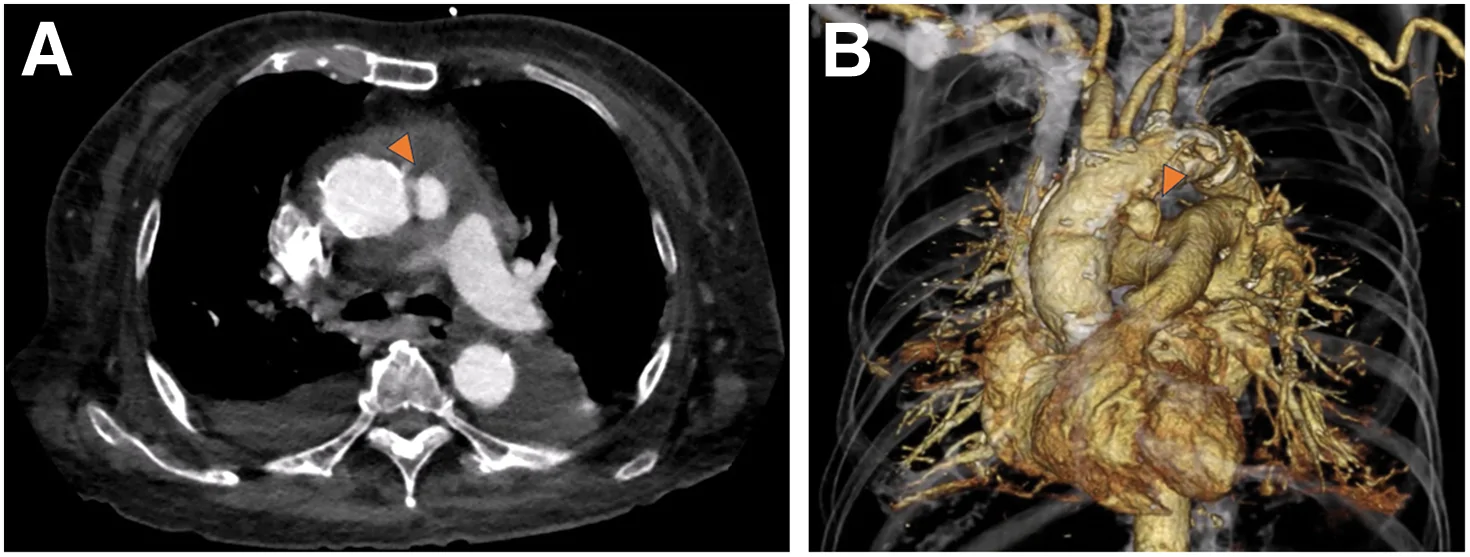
(A) Contrast-enhanced CT demonstrating an infectious pseudoaneurysm of the ascending aorta. (B) 3D CT image showing the same lesion (arrowheads).
